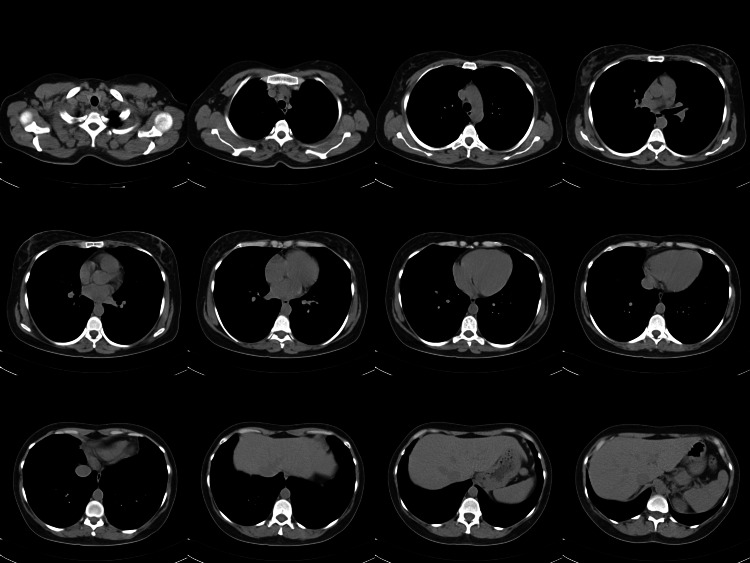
CT scan of the chest showing no remarkable findings in the lungs, mediastinum, or pleural spaces. No radiological evidence of malignancy.
